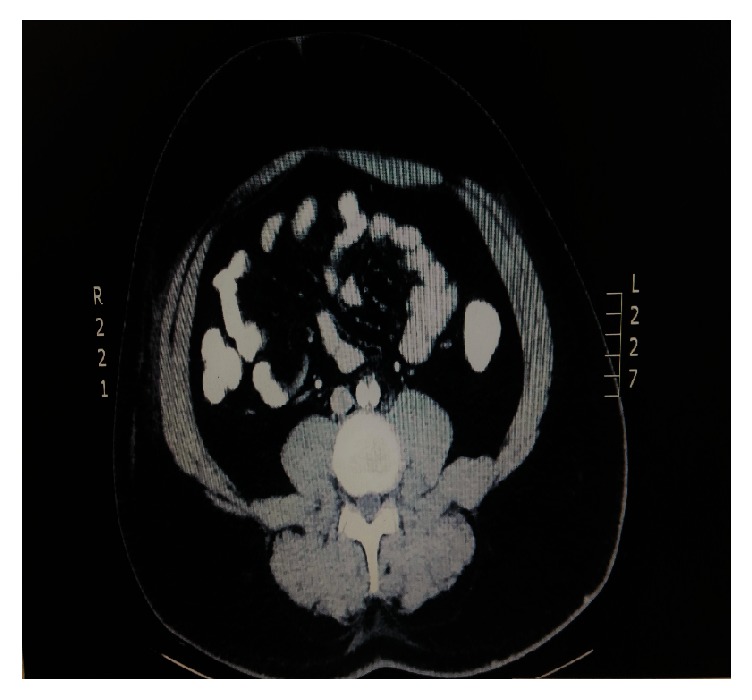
CT aortography depicted excellent patency of covered stents after 1 year.
